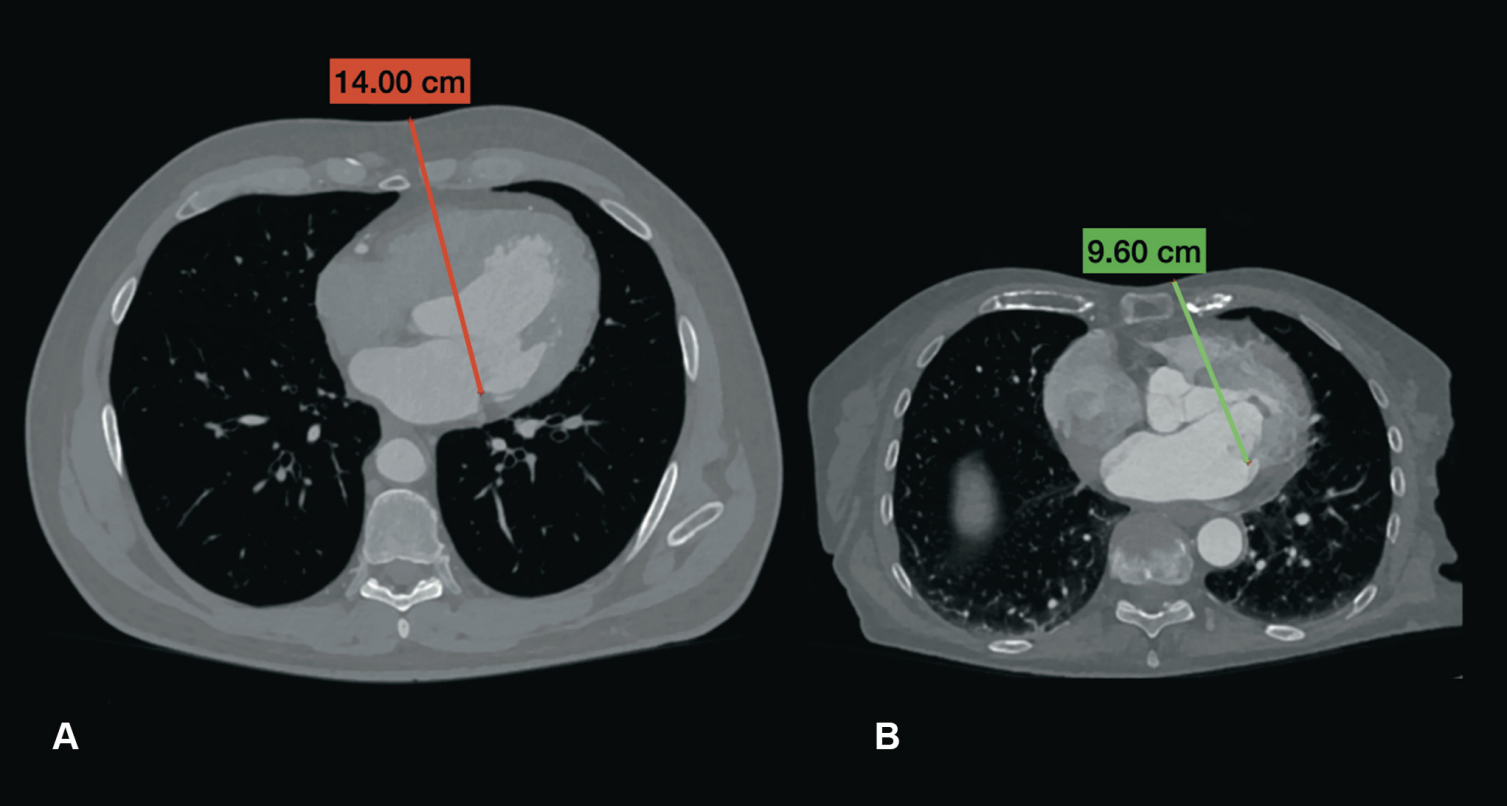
CT distance from the posterior MV annulus to the skin level determines the quality of MV exposure. A, Difficult. B, Excellent (good).
